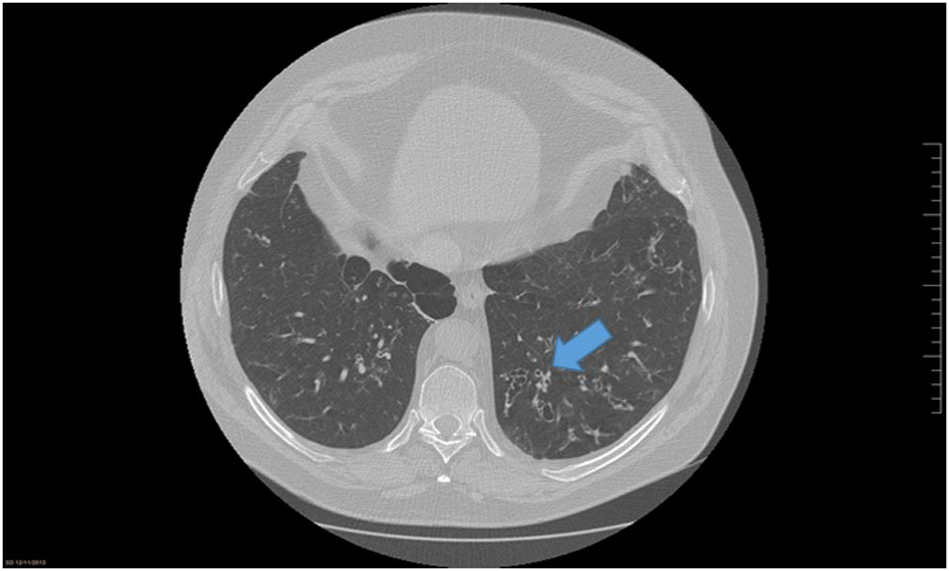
CT Ring sign in bronchiectasis. The “ring sign” is the most widely used radiological criteria for the radiological diagnosis of bronchiectasis. It is defined as a luminal diameter greater than that of the accompanying pulmonary artery.
